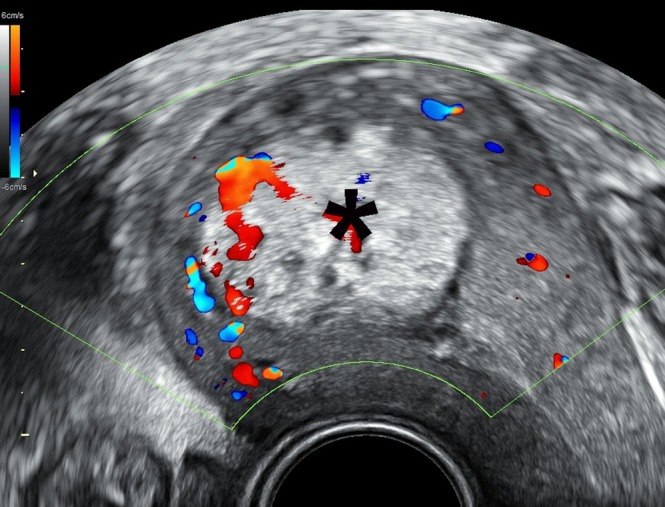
Color Doppler image mapping of retained placental tissue (
